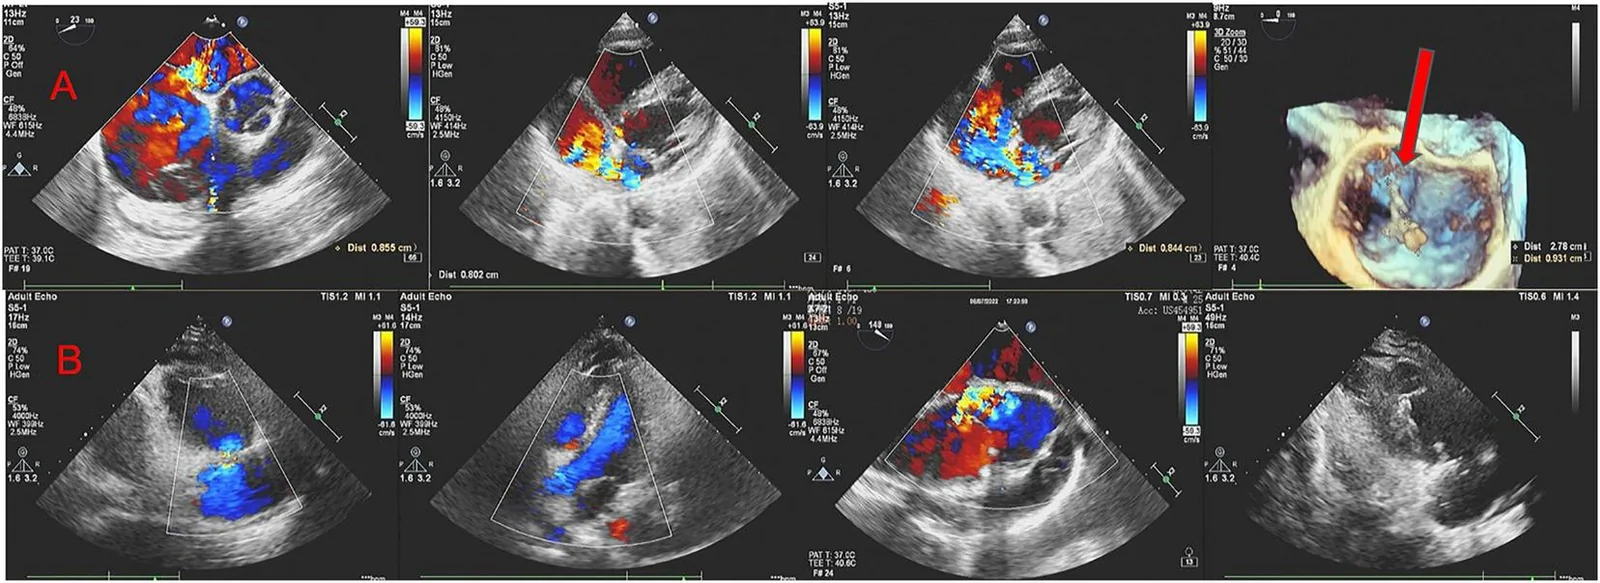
(A) Transesophageal echocardiography (TEE) revealed rupture of the tricuspid chordae tendineae with marked tricuspid regurgitation preoperatively (red arrow). (B) Three-dimensional TEE (3D-TEE) demonstrated complete resolution of the interatrial shunt and mild tricuspid regurgitation postoperatively.
